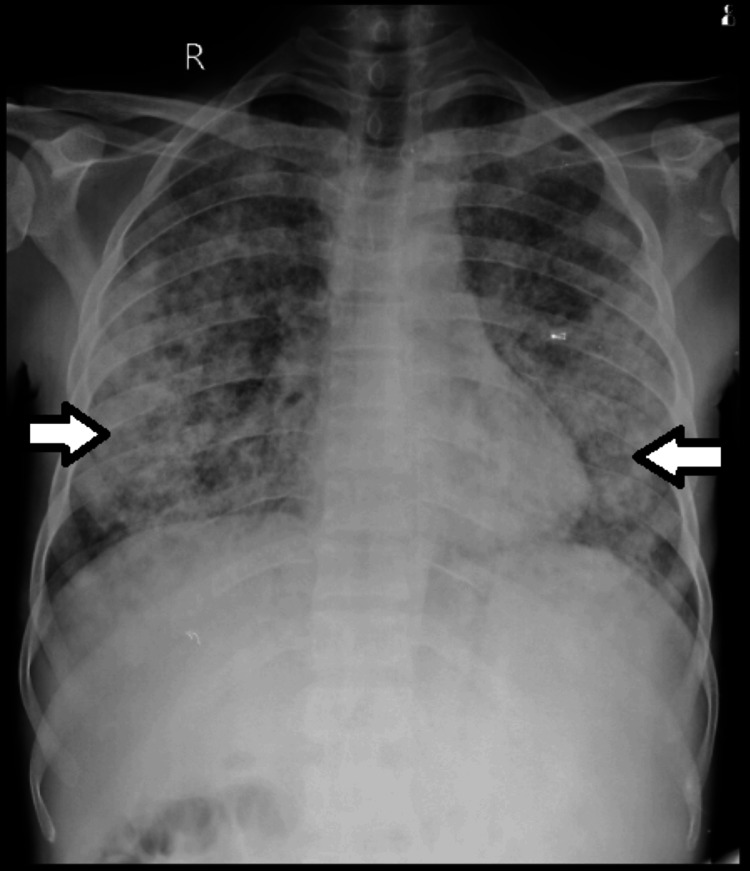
Chest X-ray of the patient showing bilateral diffuse non-homogenous nodular opacities
